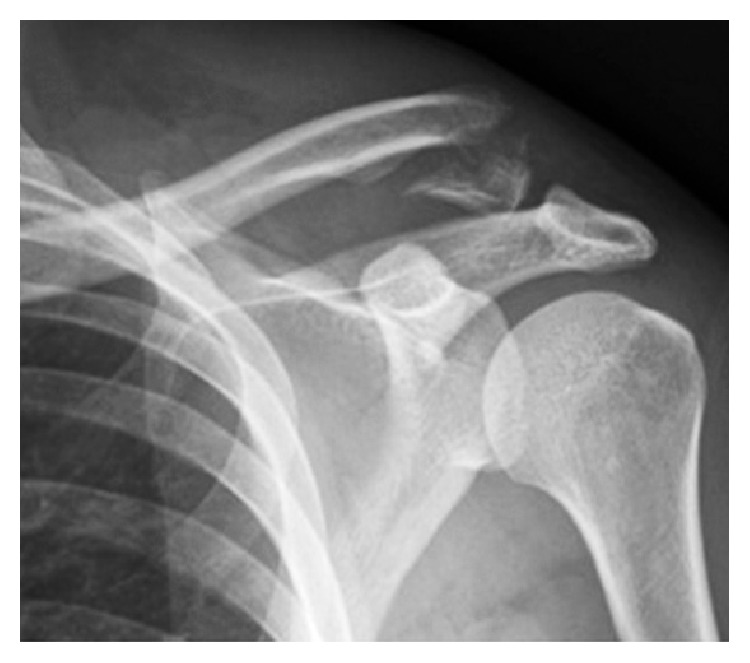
Initial radiographs prior to osteosynthesis.
